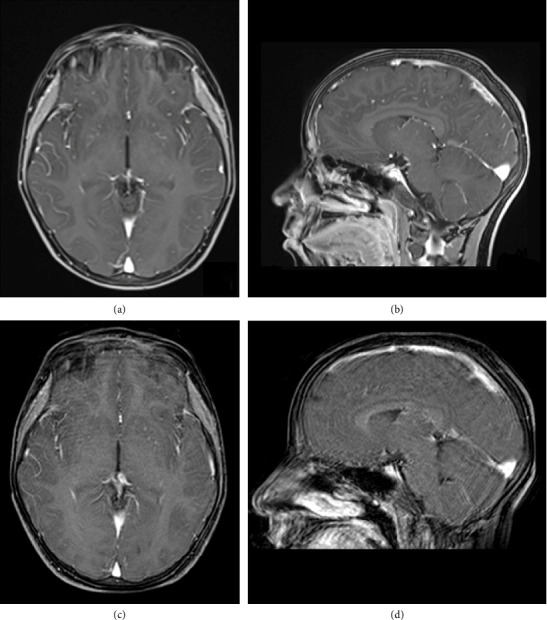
Comparison of MRI before treatment ((a) axial view and (b) sagittal view) and MRI 12 months after the start of treatment ((c) axial view and (d) sagittal view).
